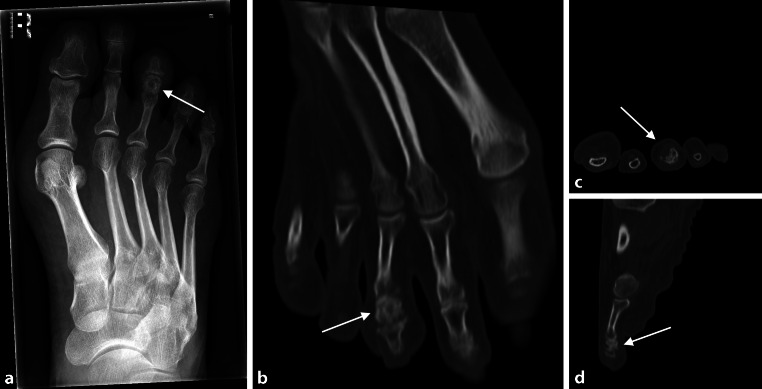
a 57-year-old female patient with osteoid osteoma of the right foot 3rd middle phalanx. X‑ray with cortical thickening and central osteolytic area occupying the entire middle phalanx of the 3rd ray (*arrows* pointing at the regions of interest). b–d Transverse (b), frontal (c), and sagittal (d) CT scans with expansive process of the distended middle phalanx, partly sclerotic, partly lytic with sporadic cortical destruction and surrounding soft tissue swelling (*arrows* pointing at the regions of interest)
